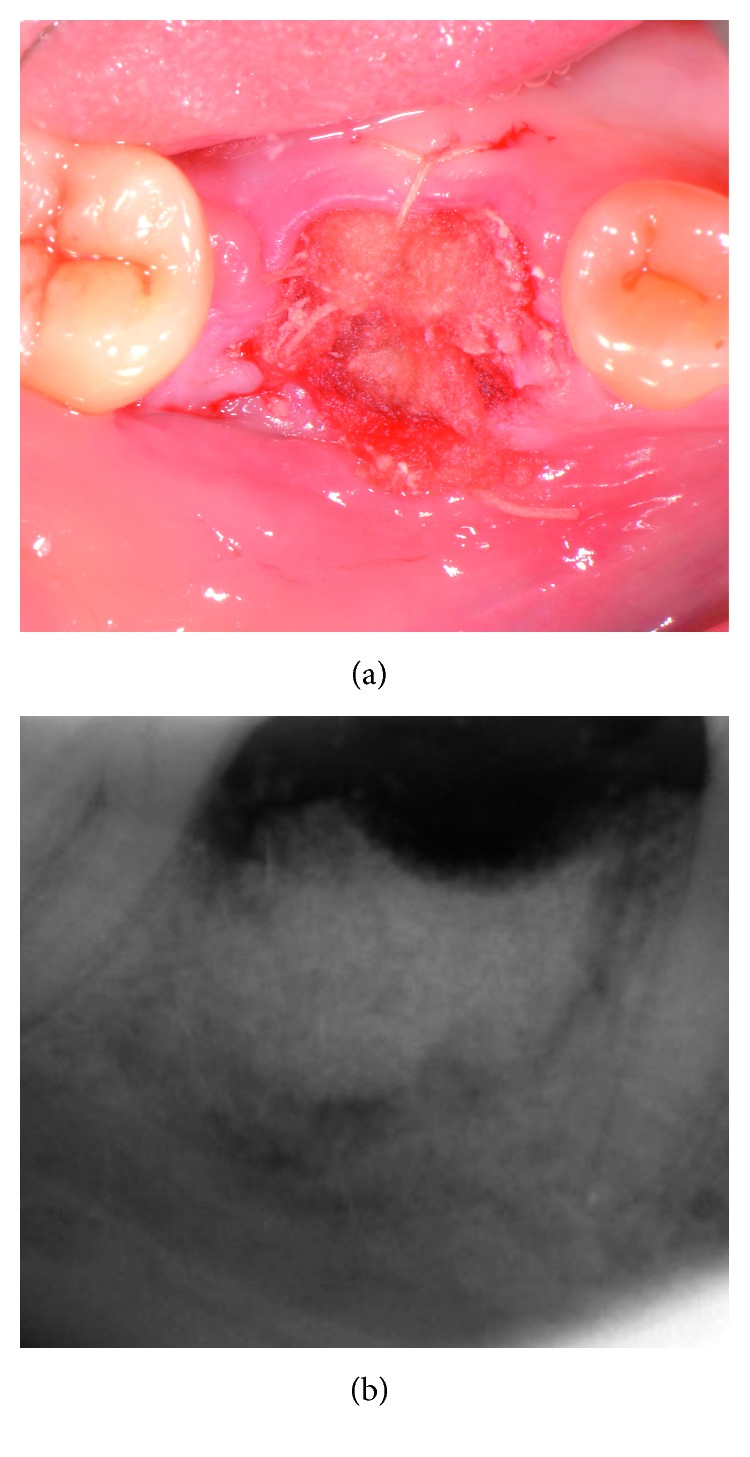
Clinical view and periapical X-ray of the grafted site.
